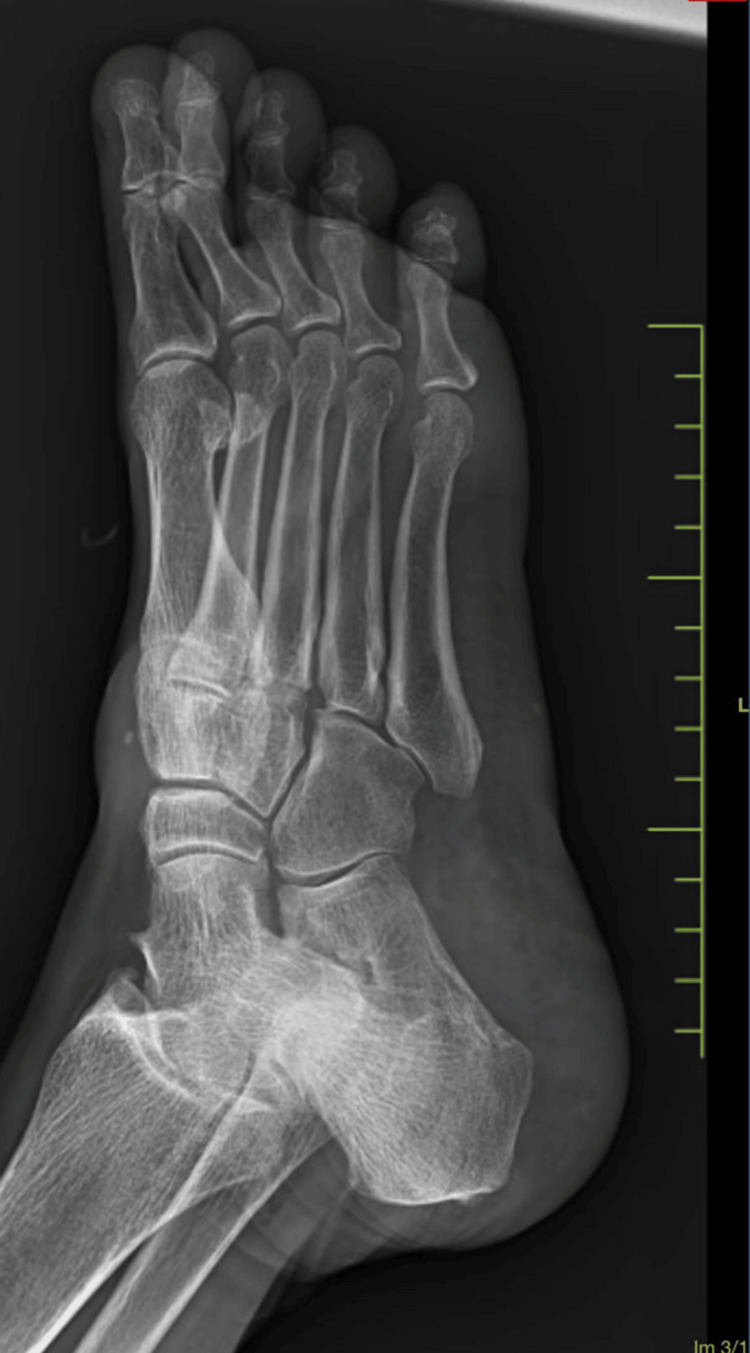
X-ray image of the foot (oblique view)
